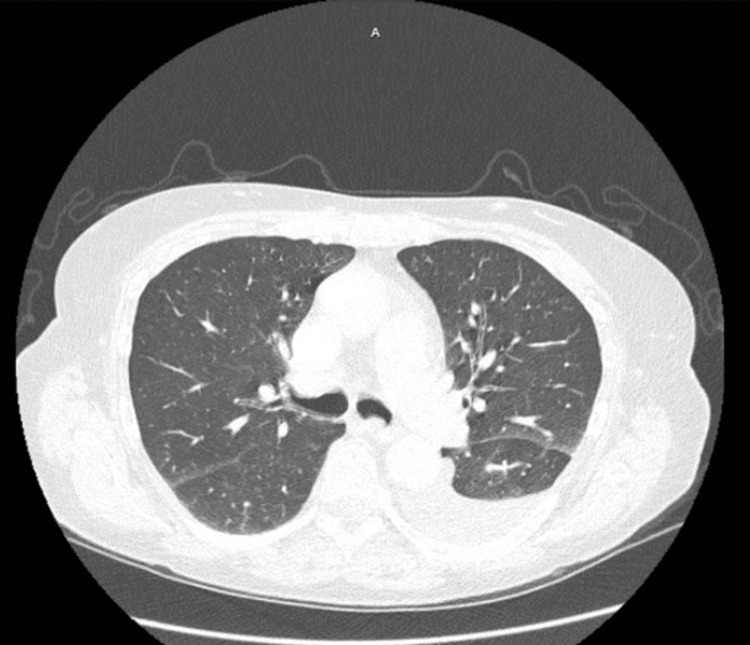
CT chest identifying left mainstem endobronchial debris which is likely a mucoid secretion
